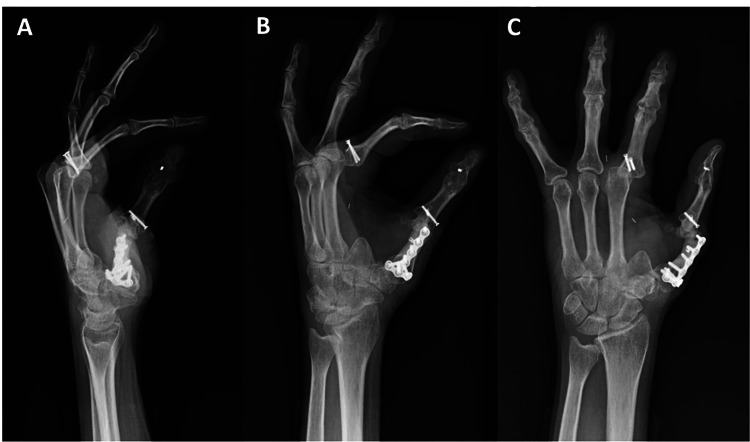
Mulitiple radiographic views (A-C) demonstrating the patient's index finger subluxation at clinic follow-up. Image Credit: Tuna Özyurekoglu
